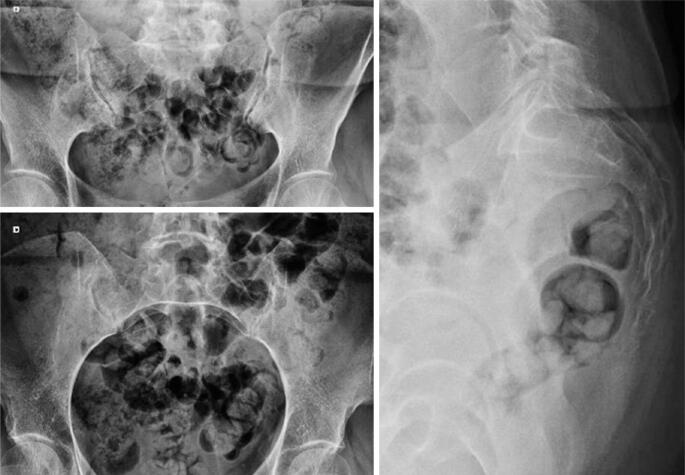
Radiograph of the pelvis and lumbar spine obtained during postpartum orthopedic evaluation. No evident signs of fracture were observed, despite persistent localized lumbosacral pain
